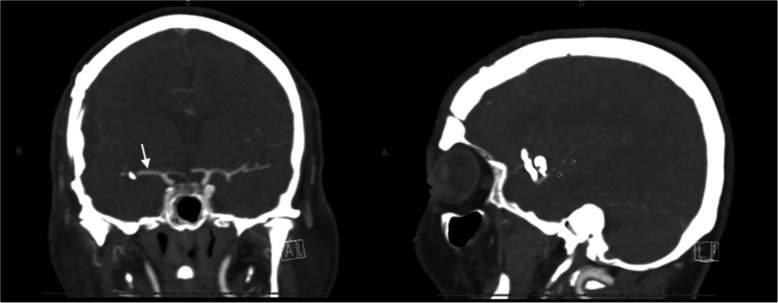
CT angiogram on Day-0 of acute left hemiparesis. Left (white arrow) showing a patent M-1 segment of the right MCA and Right (Dashed arrows) showing spastic M2 segment of the right MCA
